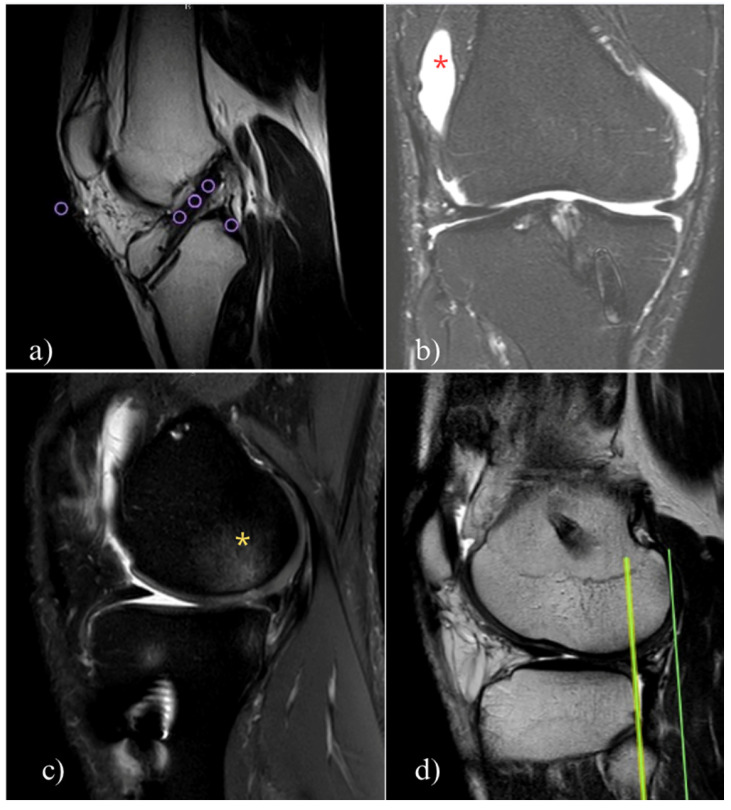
Common direct signal of graft maturity in MRI. (a) The signal–noise quotient is calculated by averaging the signal intensity from the distal, middle, and proximal grafts, subtracting the signal from the posterior cruciate ligament, and dividing it by the background signal. The purple circles indicate the region of interest for detecting the signal intensity. (b) Intra-articular swelling; the red asterisk indicates swelling. (c) Bone bruise of the posterior lateral femoral condyle; the yellow asterisk indicates the bone bruise in the posterior lateral femoral condyle. (d) Posterior femoral translation relative to the tibia; the dark green line indicates the femoral position relative to the tibial position (light green line).
